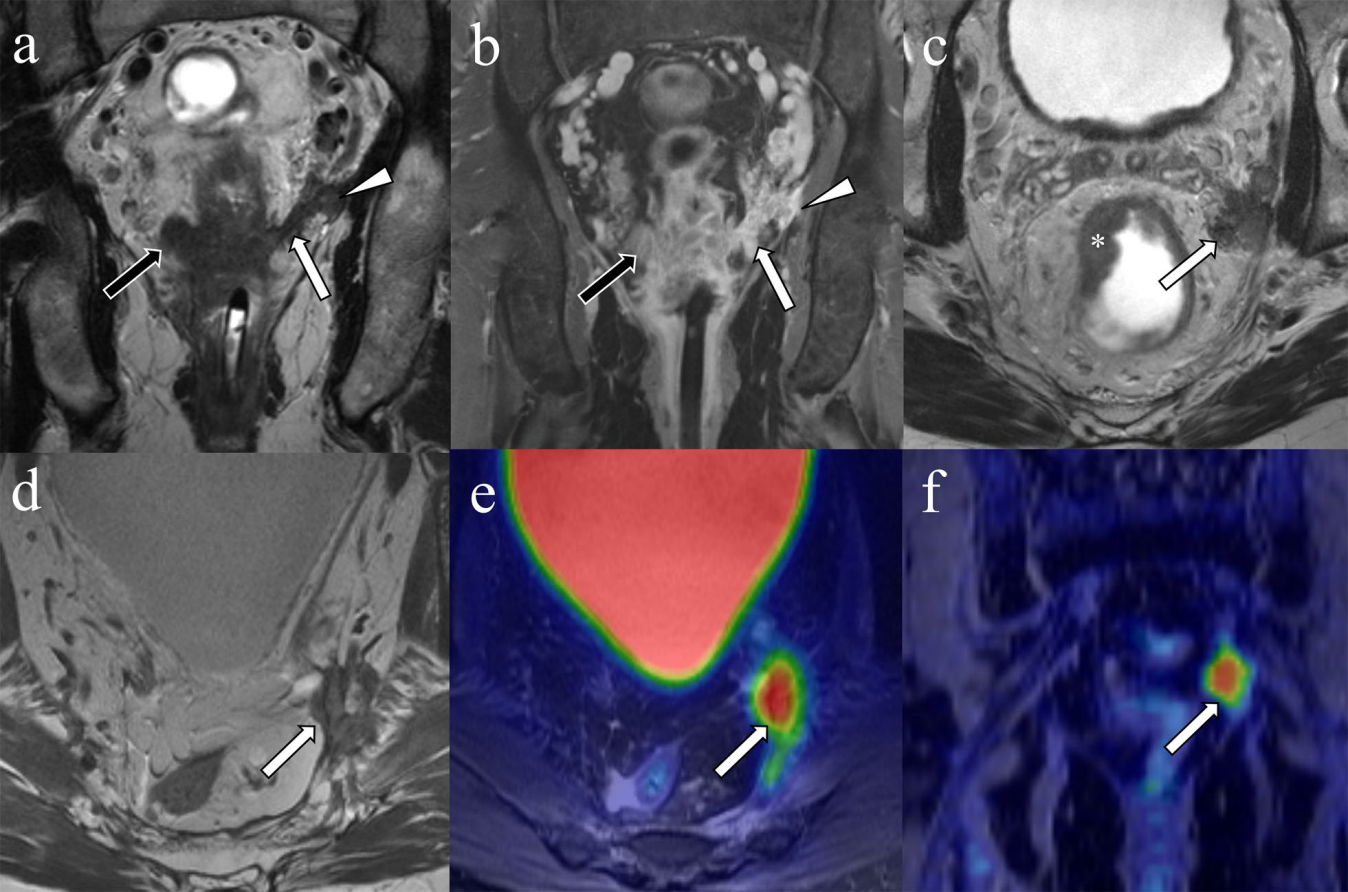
Local recurrence after surgery from residual disease associated with positive circumferential margin due to extramural venous invasion. A 58-year-old man with rectal cancer. T2-weighted (a) and postcontrast (b) coronal image before treatment demonstrate nodular-shaped structure (black arrows) on the right extending to the mesorectal fat tissue, and worm-shaped (white arrows) structure on the left with tumor signal intensity arising from the primary lesion, indicating extramural venous invasion (EMVI) extending the mesorectal fascia (arrow heads). Axial T2-weighted image shows a primary tumor (c asterisk) and an irregular tumoral deposit in and abutting the mesorectal facia near the left pelvic sidewall (c arrow). Axial and coronal ^18^F-FDG-PET/MRI images 23 months after surgery following neoadjuvant therapy show FDG avidity corresponding to a developed nodular recurrence at the same location (d–f arrows)
